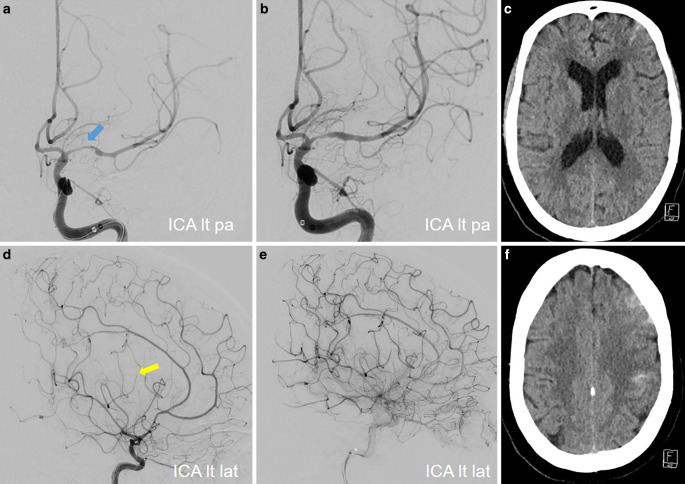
Endovascular treatment of the severe intracranial stenosis with non-occlusive thrombus in the proximal left M1 segment. Diagnostic angiograms of the left ICA (PA view, a; lateral view, d) demonstrating severe stenosis of the left M1 segment with adjacent thrombus (blue arrow), distal perfusion delay and a peripheral embolus in a precentral branch (yellow arrow). Angiograms after stent implantation (PA view, b; lateral view, e) showed complete coverage of the lesion with an improved distal perfusion. Native CT scan on day 1 after treatment (axial views; c, f) demonstrated minimal SAH in the sulci of the left frontal and parietal lobes and no new cerebral infarction
